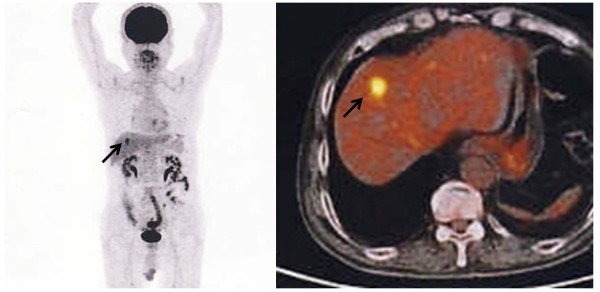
^18^Fluorine-fluorodeoxyglucose positron emission tomography (FDG-PET) findings. FDG-PET showed strong uptake in the liver (arrow). No other abnormal uptake was observed.
